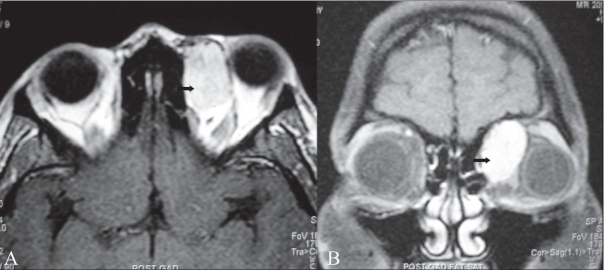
Post-contrast axial (A) and coronal (B) T1W MRI images shows intense enhancement of the lesion (arrows). Inferolateral displacement of the globe is well seen on the coronal image (B)
